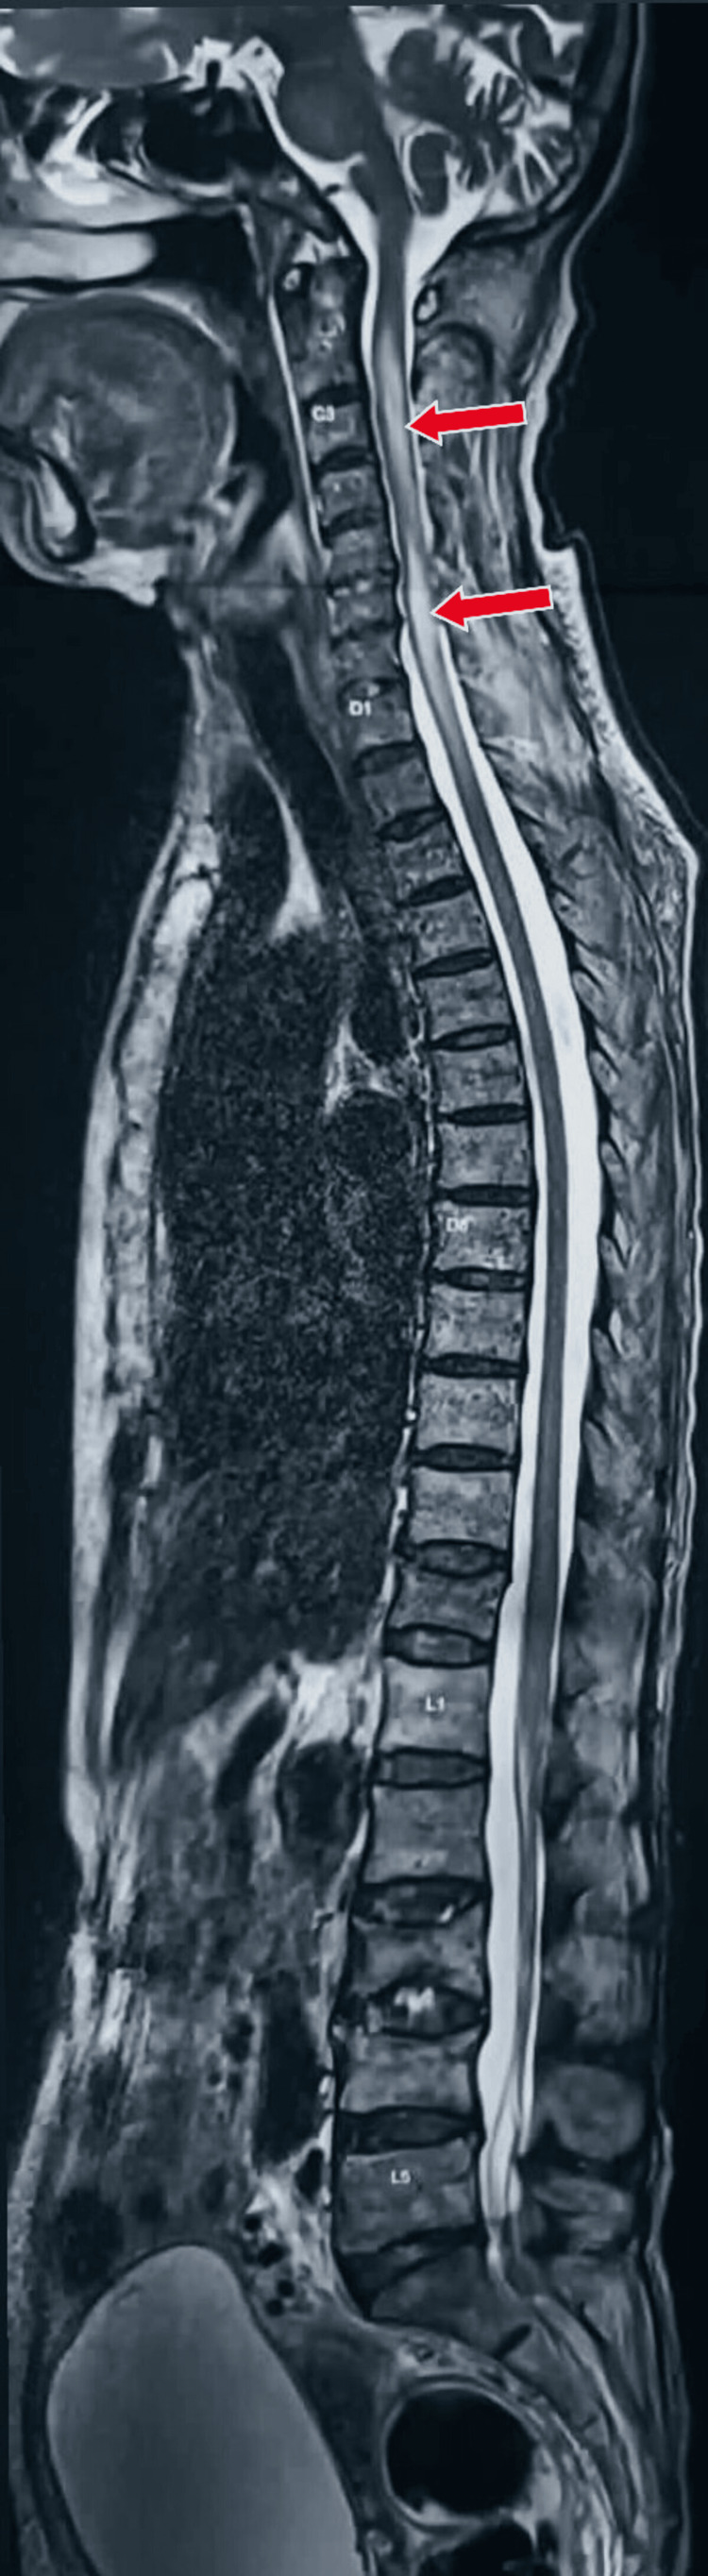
MRI whole spine screening (T2 sagittal section) showing longitudinal extensive lesion of spinal cord at C2-D1 level with central cord prominence marked with red arrows. MRI: magnetic resonance imaging.
